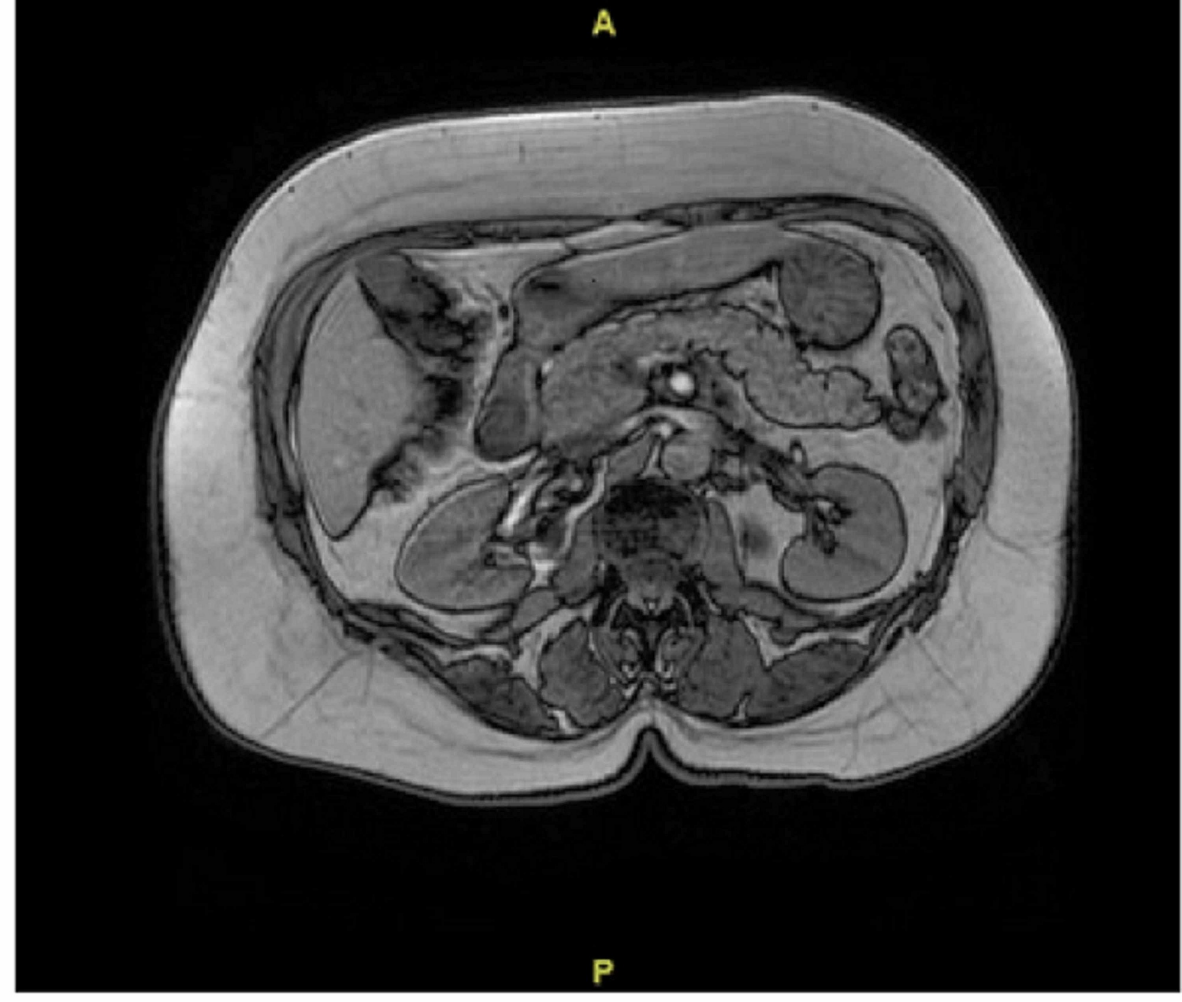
Magnetic resonance imaging/magnetic resonance cholangiopancreatography (MRI/MRCP): reporting mild enhancement and edema around the head and uncinate process of the pancreas and around the body and tail suggestive of pancreatitis.
